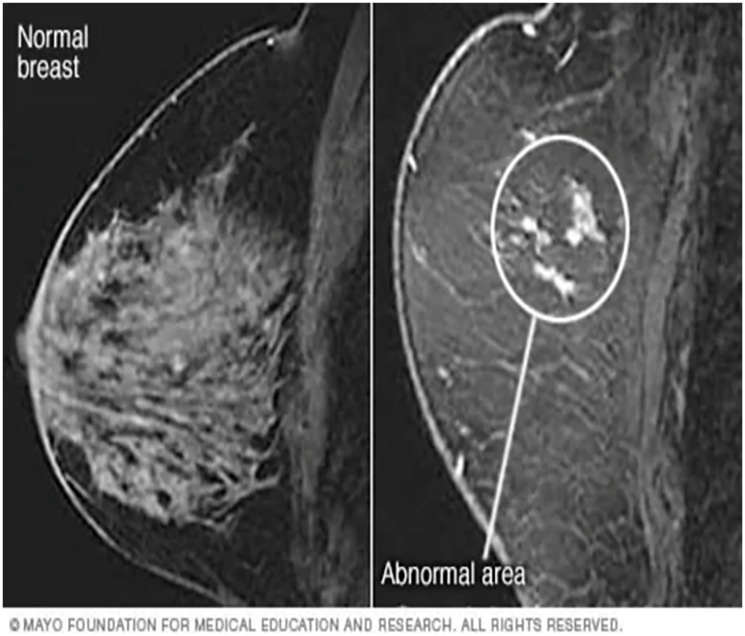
Difference between MRI scan of normal breast versus abnormal [28].
